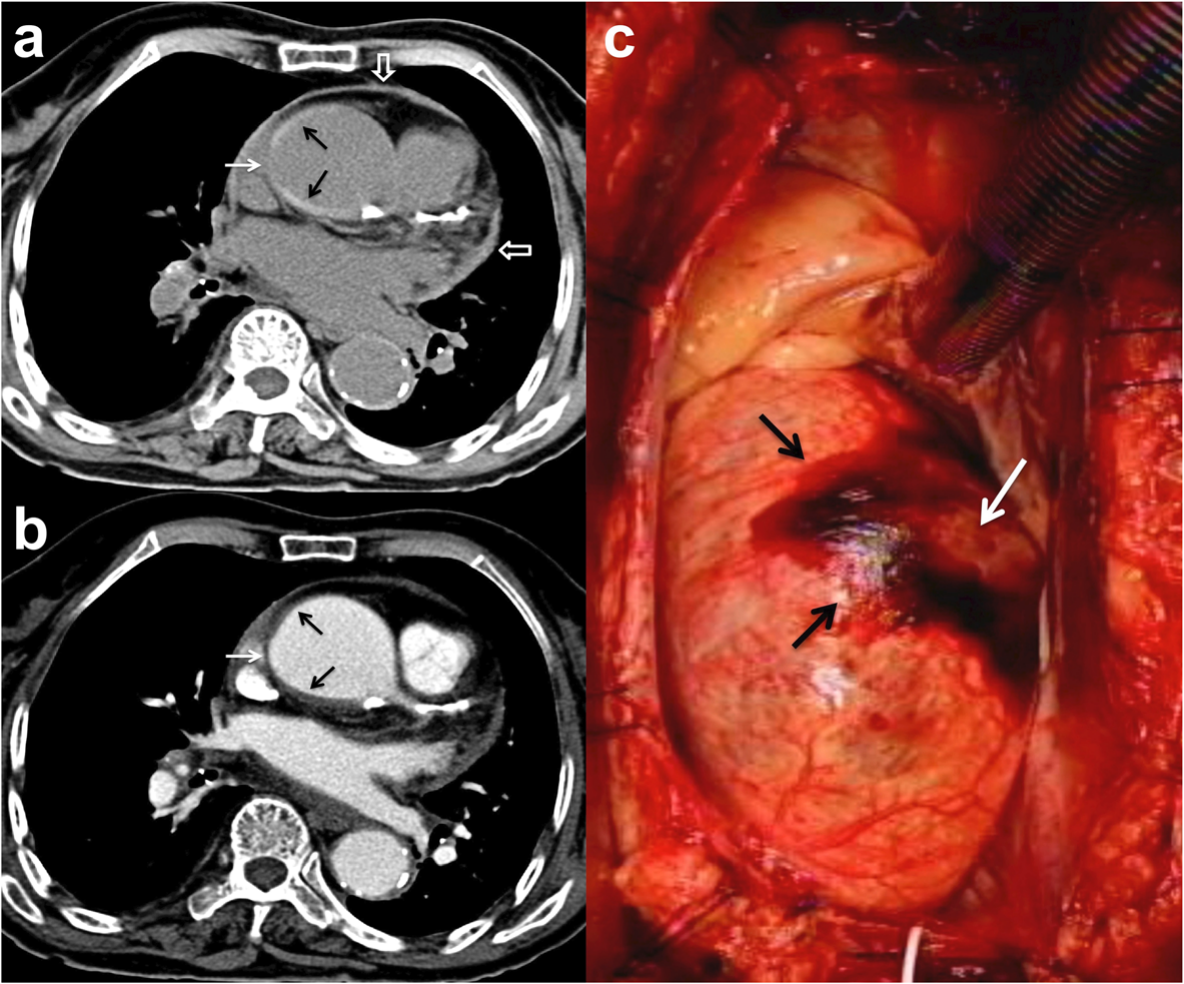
a Unenhanced axial CT shows a smooth central defect (white arrow) in the hyper-attenuating crescentic hematoma (black arrows) along the ascending thoracic aorta and minimal hemopericardium. b Enhanced CT shows a smooth focal out-bulging (white arrow) of the aortic lumen corresponding to the defect on unenhanced study and non-enhancing crescentic hematoma (black arrows). Note the absence of intimal flap or contrast medium extravasation. c Surgical findings confirmed ecchymosis at the ascending aorta caused by IMH and focal aortic adventitial defect
